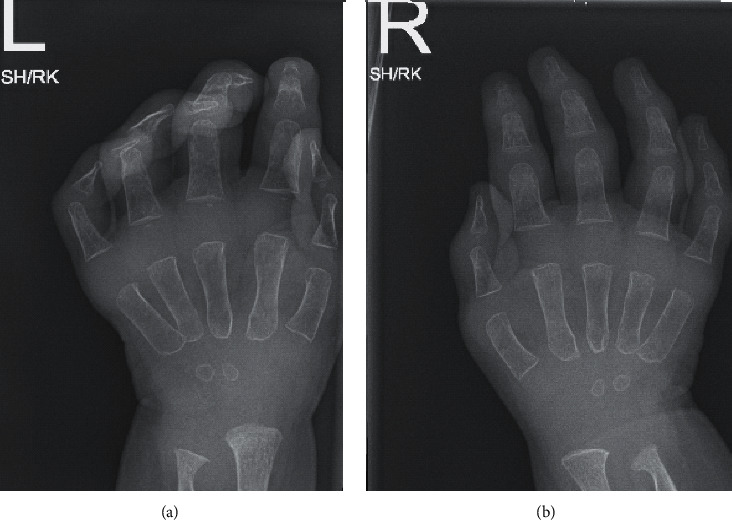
X-ray of the patient's hands (left: labelled L and right: labelled R) showing soft tissue swelling of both distal hands with grossly maintained joint spaces.
